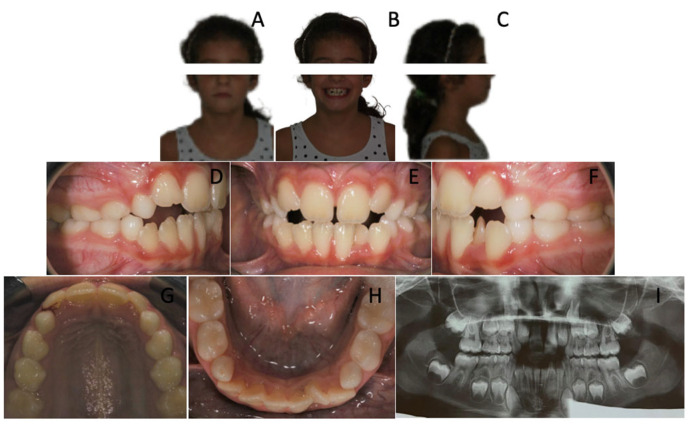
A 7-year-old female patient with two supplemental mandibular teeth. (A–C) Extra-oral photopraphs; (D–H) Intra-oral photographs; (I) Orthopantomography.
